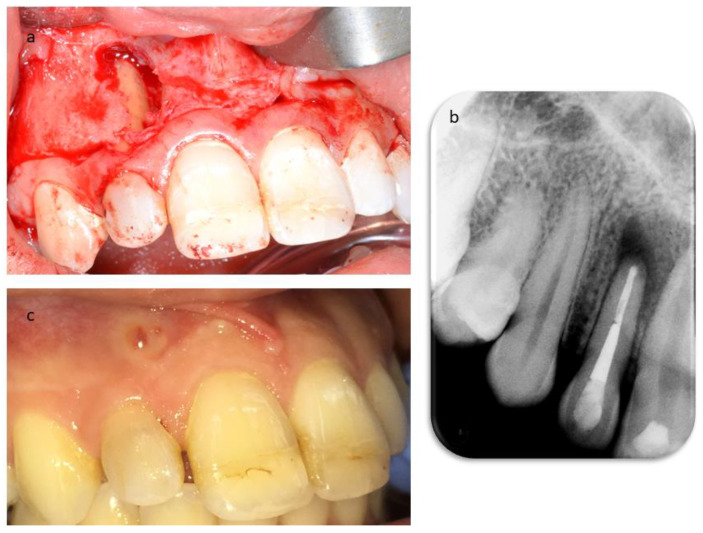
(a) Intra-operative picture of the surgical endodontic treatment. (b) Post-operative periapical radiograph. (c) Clinical examination of the patient at one month after surgery showing the persistence of the stoma.
